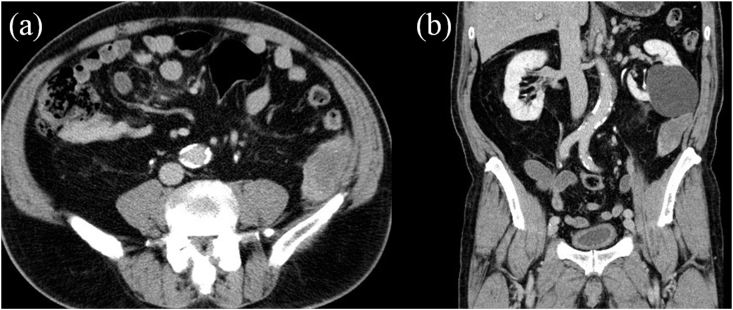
Abdominal CT images (a: axial, b: coronal view) showed a 7-cm-diameter spindle shaped lesion just inferior to the left renal cyst. The mass showed a slight contrast effect.
